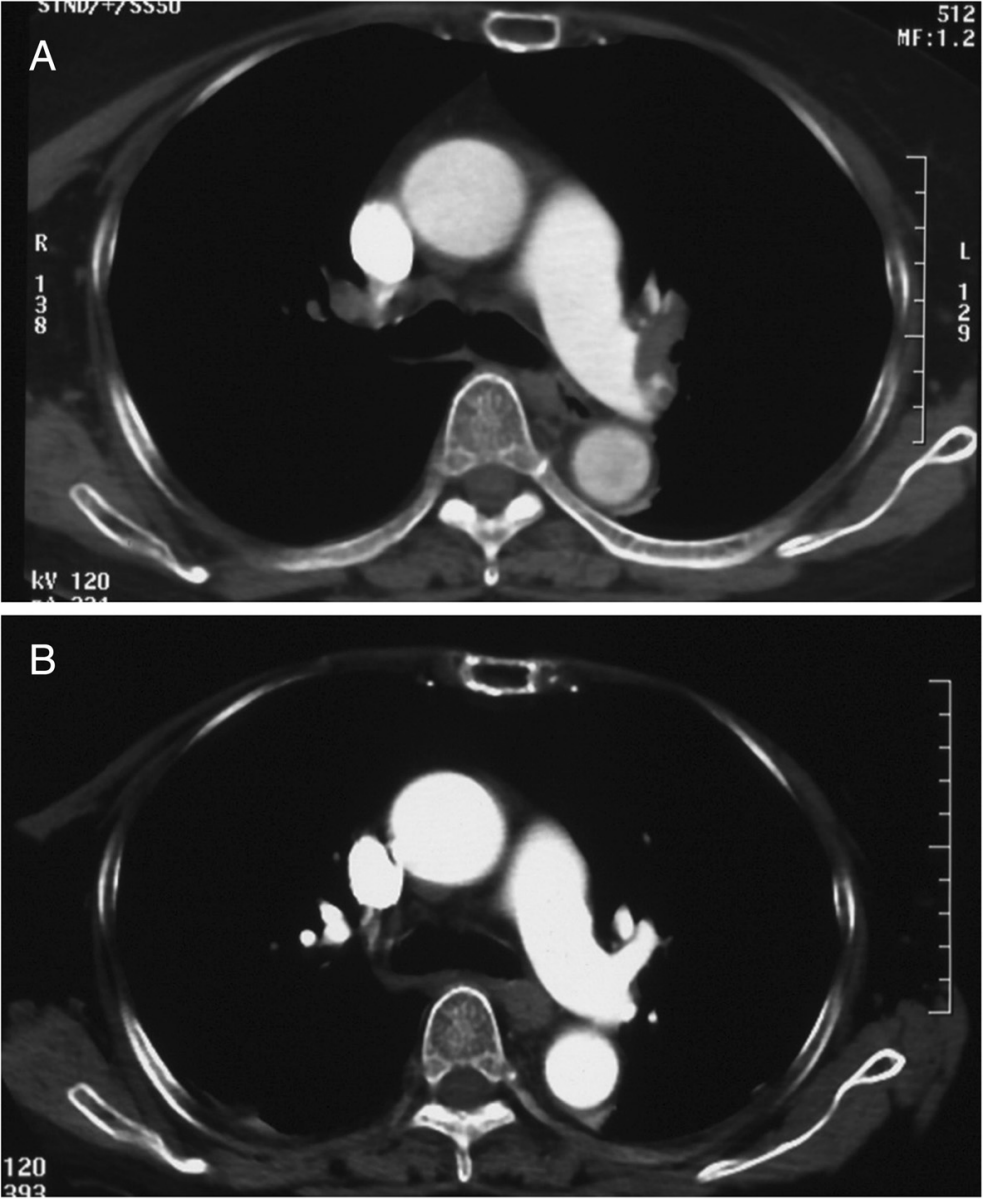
Pulmonary thromboembolism on computed tomography (CT) scan. A: CT scan showed thrombosis in the bilateral main pulmonary arteries. B: Disappered thrombosis in the bilateral main pulmonary arteries.
